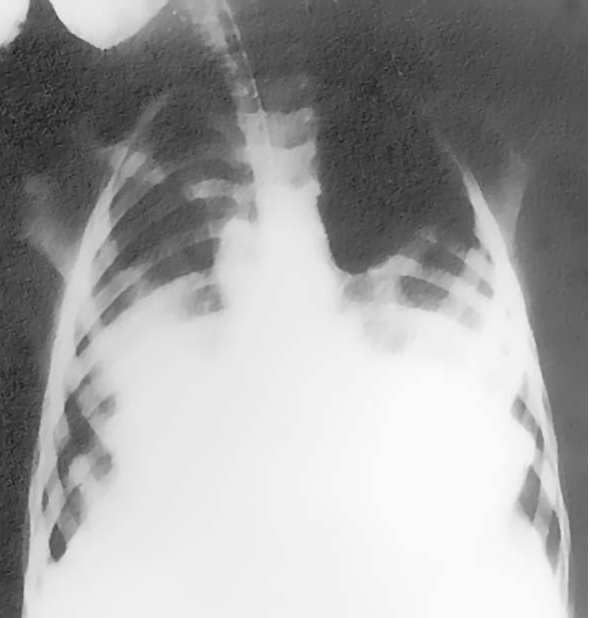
Chest roentgenogram upon admission. Frontal chest roentgenogram revealed extensive bilateral diffuse pulmonary infiltrations, left greater than right.
